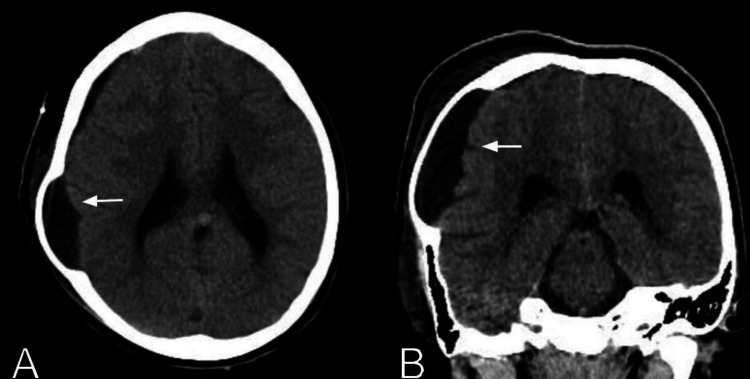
Postoperative head CT scan axial (A) and coronal (B) views showing complete drainage of subdural hematoma and intracystic hemorrhage, demonstrating the cerebrospinal fluid-filled parietal arachnoid cyst (white arrows).
